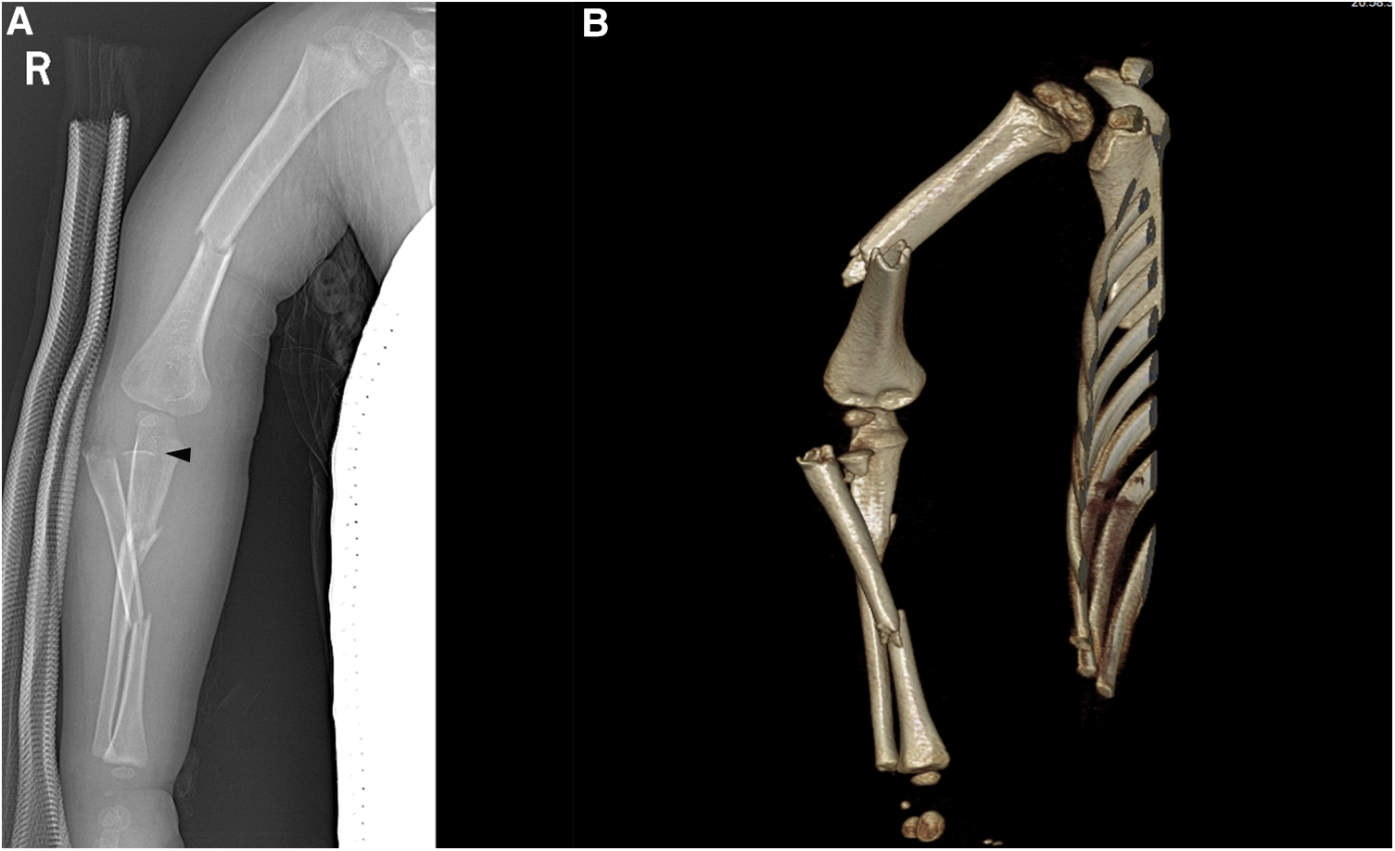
x-ray (A) and CT (B) images of the injured child at the time of injury; the black triangle represents the unossified radial head.
